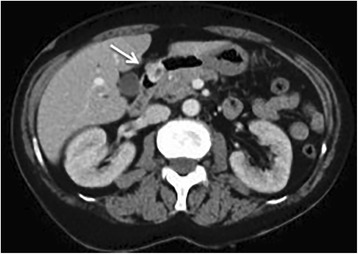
A tumor with contrast effect found (on abdominal contrast CT scan) to protrude into the duodenal cavity
